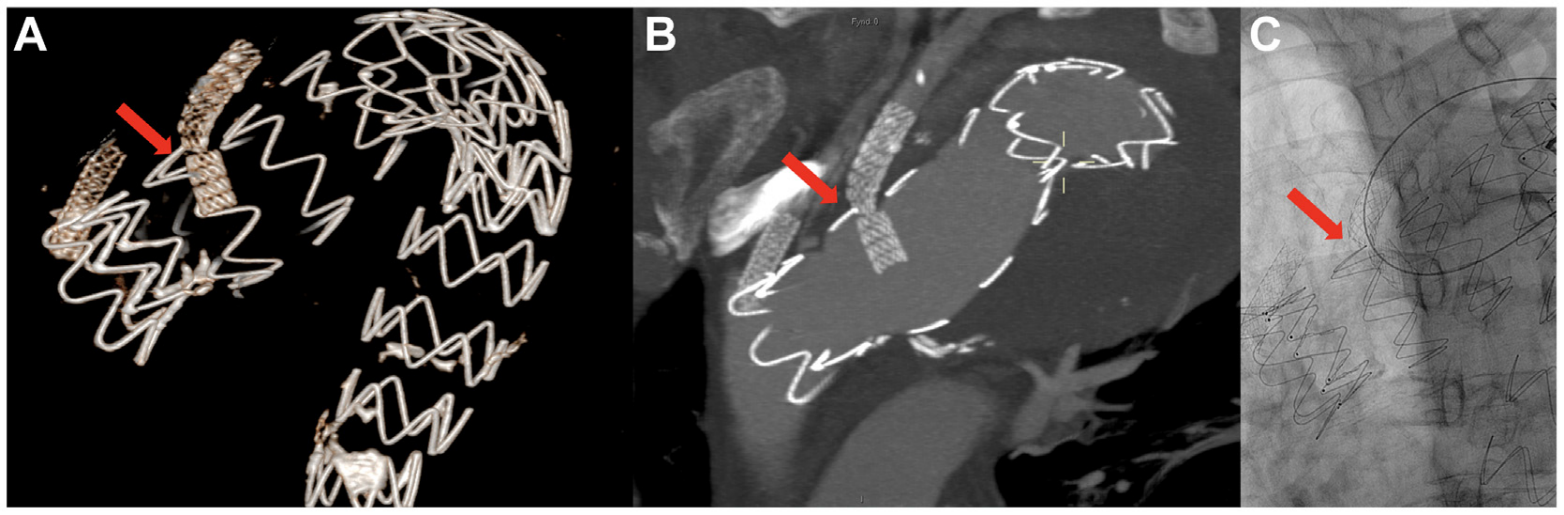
A, Three-dimensional reconstruction of the fractured left subclavian artery (*LSA*) bridging stent (*red arrow*). B, Two-month computed tomography (*CT*) scan showing significant stenosis of the LSA bridging stent graft (*red arrow*). C, Intraoperative image of the fractured bridging stent graft (*red arrow*).
